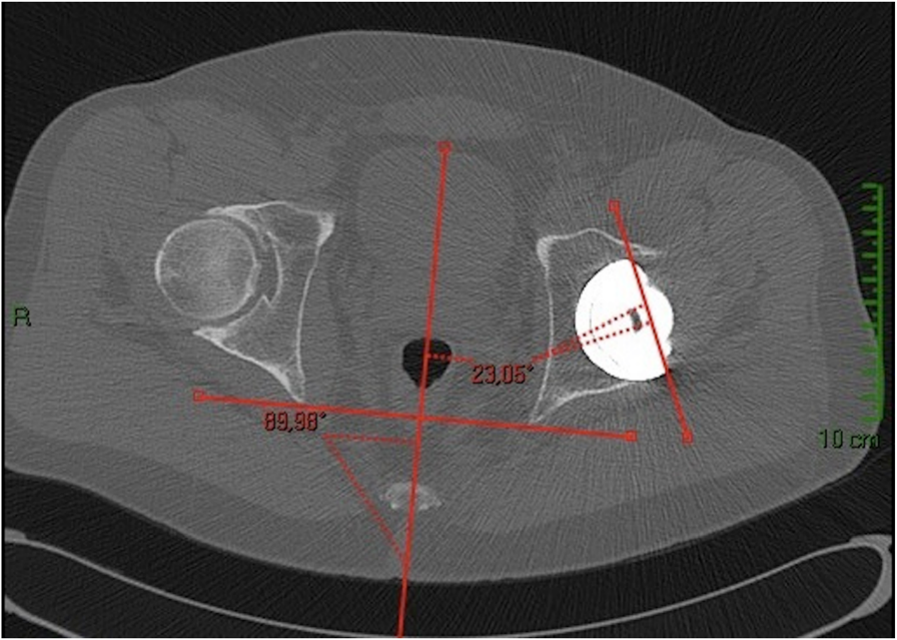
CT-scan showing cup anteversion of 23°
